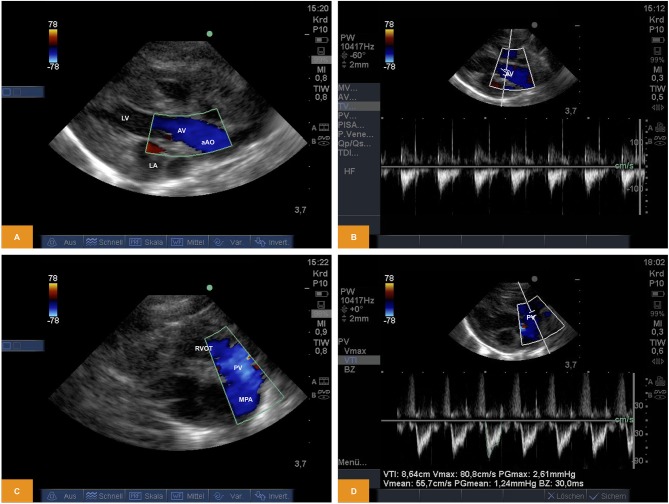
(A,B) Doppler study of flow across the aortic valve. The image was obtained from PLAX. Note the in favorable angle of insonation for measurement of pulsed wave Doppler (age: 70 h, weight: 1.45 kg). (C,D) Doppler study of flow across the pulmonary valve. The image was obtained from PSAX (age: 70 h, weight: 1.45 kg). aAo, ascending aorta; AV, aortic valve; LA, left atrium; LV, left ventricle; MPA, main pulmonary artery; PV, pulmonary valve; PLAX, parasternal long axis; PSAX, parasternal short axis; RV, right ventricle; RVOT, right ventricular outflow tract.
